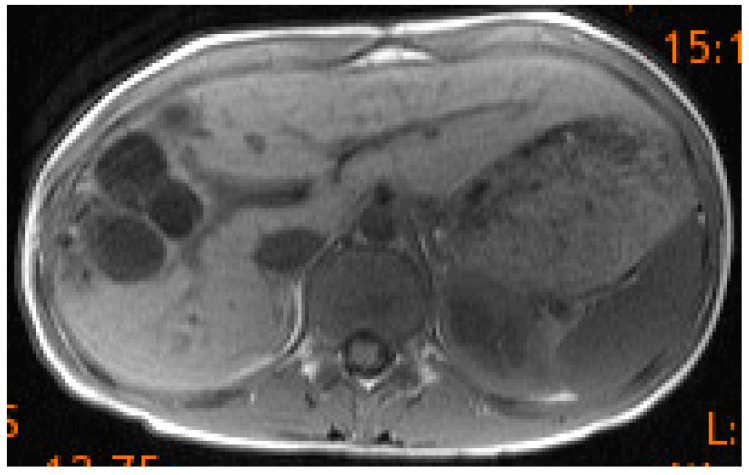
CT of the upper abdomen detecting recurrent cysts after surgery on a traumatically ruptured EC in the seg. 5/6.
